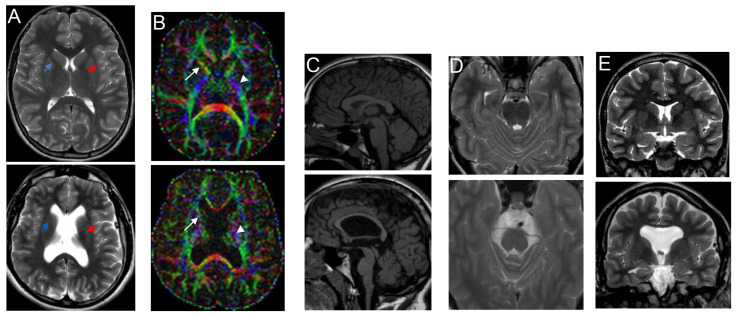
Brain MRI scans of the 17-year-old patient (bottom panel) compared to an age-matched control (upper panel): (A) transverse T2 SE, (B) color fractional anisotropy, (C) sagittal T1 SE, (D) transverse T2 SE, (E) coronal T2 SE. (A) Lateral ventricle enlargement with dysmorphic appearance; the dysmorphic and hypoplasic anterior limb of the internal capsule (light blue arrow) with partial fusion of lenticular nucleus and caudate nucleus head (red arrow). (B) Thinning of the corticospinal tract is evident at the level of the posterior arm of the internal capsule (white head arrow). (C) Thinned and dysplasic corpus callosum. (C,D) Pontomesemcephalic hypoplasia with defect of segmentation and dysmorphic appearance. (E) Septum pellucidum agenesis, thickened and dysmorphic fornices, anterior commissure agenesis, optic chiasm hypoplasia. (A,E) Simplified pattern of gyration of the frontal lobes with global volumetric reduction.
